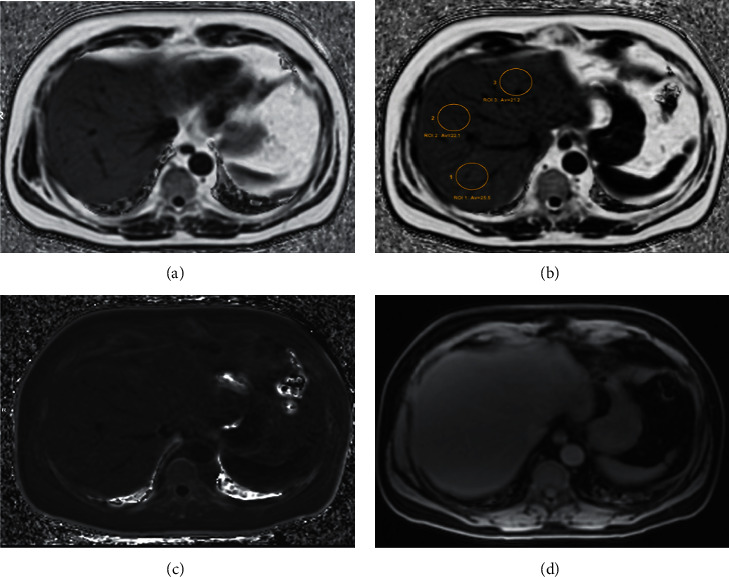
Fat fraction MRI images of a 65-year old male patient with NAFLD. (a) MRI-PDFF: 3D Ax IDEAL-IQ image. (b) MRI-PDFF: 3D Ax IDEAL-IQ image with ROIs placed showing high values of fat deposition than normal liver (>5%). (c) R2^∗^ 3D Ax IDEAL-IQ image representing the T2^∗^ correction needed to estimate MRI-PDFF. (d) Water 3D Ax IDEAL-IQ image as expression of different echo times used for the IDEAL-IQ sequence acquisition. MRI-PDFF: magnetic resonance-fat fraction of the estimated proton density; echo asymmetry and least squares estimation (IDEAL-IQ); NAFLD: nonalcoholic fatty liver disease; ROIs: regions of interest.
